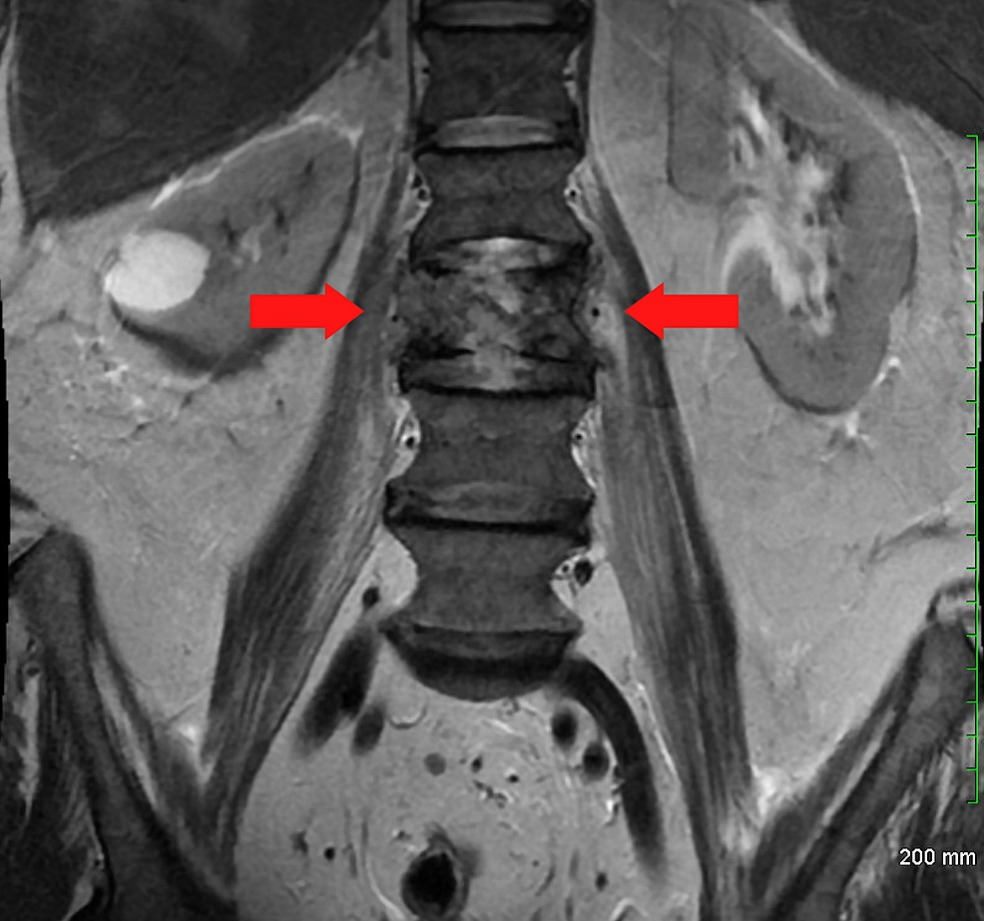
MRI of lumbar spine. Aggressive marrow replacing a mass of the L3 vertebral body causing focal severe spinal canal narrowing. Pathology showing metastatic poorly differentiated carcinoma. Red arrows indicate the mass effect in the vertebral body as a result of metastatic disease.
